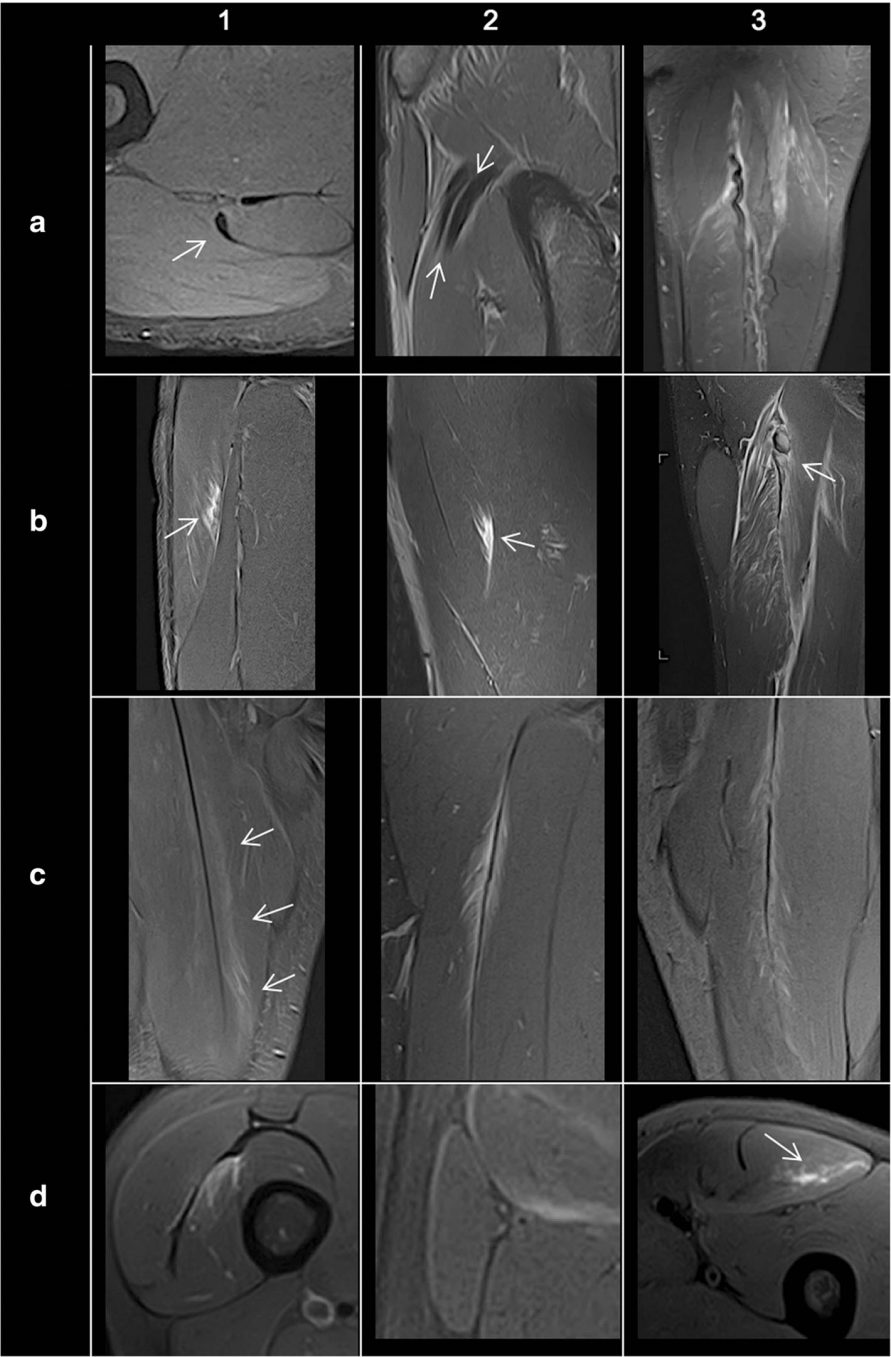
MRI findings and patterns in acute muscle injuries of the lower limb. Tendon injuries showing elongation without a measurable tear (a1), longitudinal tear (a2), and total transverse tear with marked tendon retraction (a3). Peripheral myoaponeurotic/myofascial/myotendinous injuries showing intact aponeurosis (b1), fascial tear (b2), and tendinous complete section with hematoma and retraction (b3). Central myotendinous (septal) injuries showing intact septum (c1), fiber gap of the septum (c2), and total septal tear with loss of tension (c3). Injuries of isolated muscle fibers without disruption (d1) with architectural distortion or blurring (d2), or with measurable tear (d3)
